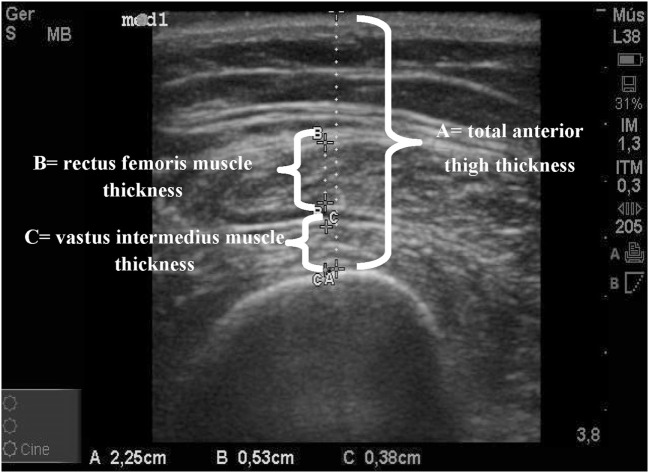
Ultrasound scan with measurements of the dominant thigh. A = total anterior thigh thickness in centimeters; B = rectus femoris muscle thickness in centimeters; C = vastus intermedius muscle thickness in centimeters; B + C = quadriceps muscle thickness in centimeters (attached).
